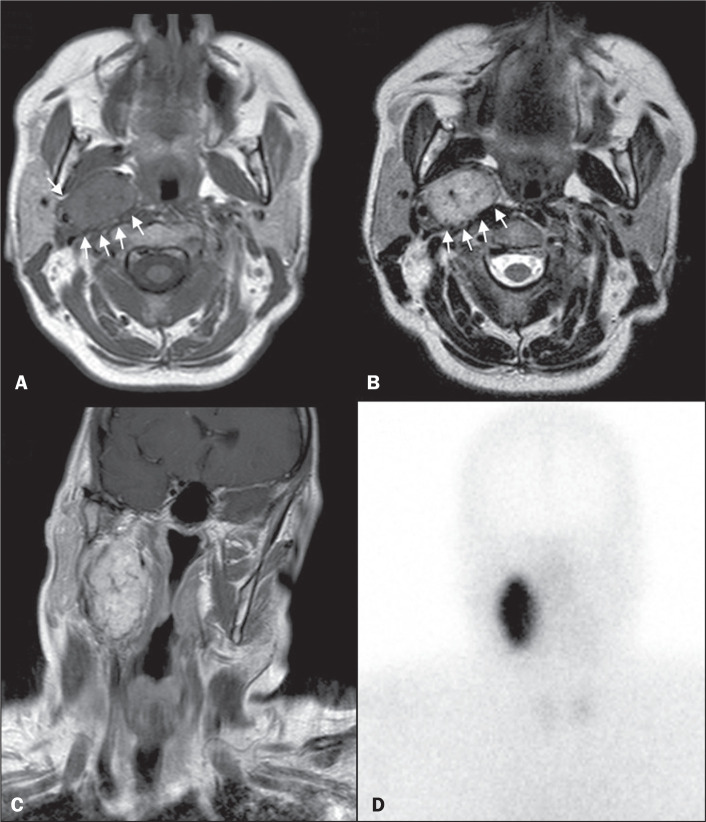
MRI and planar SRS images of patient A5, who received a confirmed
